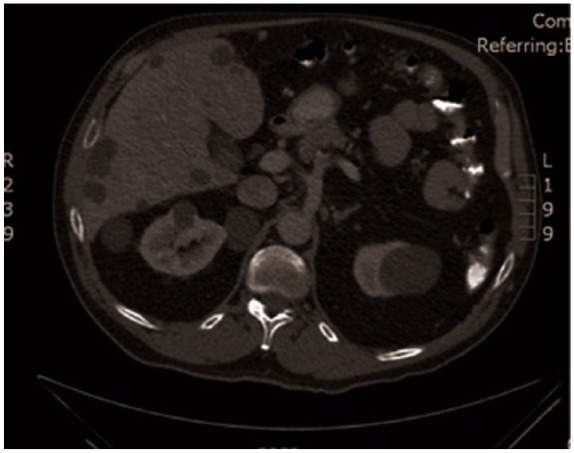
CT abdomen/pelvis with contrast 3 months prior to presentation, obtained in the setting of diverticular disease. Innumerable low-attenuation liver and multiple kidney cysts noted previously.
